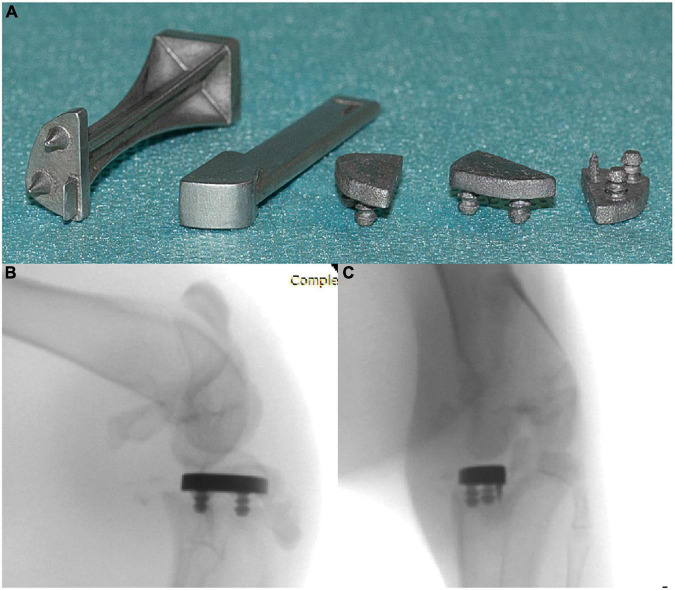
(A) Ti-6Al-4V implants (right) and instruments (left). Post-mortem radiographs showing the position of the implant in AP (B) and lateral (C) views.
